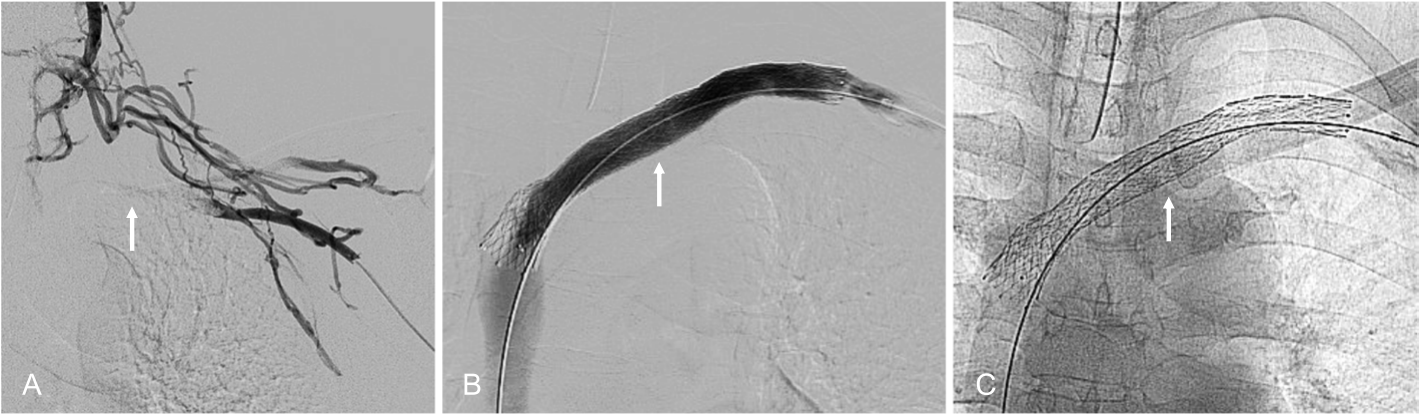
50-year-old man with recurrent left axillosubclavian venous thrombosis and left upper extremity pain and swellin*g (A) Left upper extremity venography demonstrating left axillosubclavian venous occlusion (arrow) (B) Left upper extremity venography, after left axillosubclavian stent reconstruction using 14-mm Venovo Venous Stents (arrow), showing brisk in-line from the left upper extremities to the right atrium (C) Radiograph demonstrating the 14-mm Venovo stent in the thoracic outlet (arrow) without deformation or fracture
